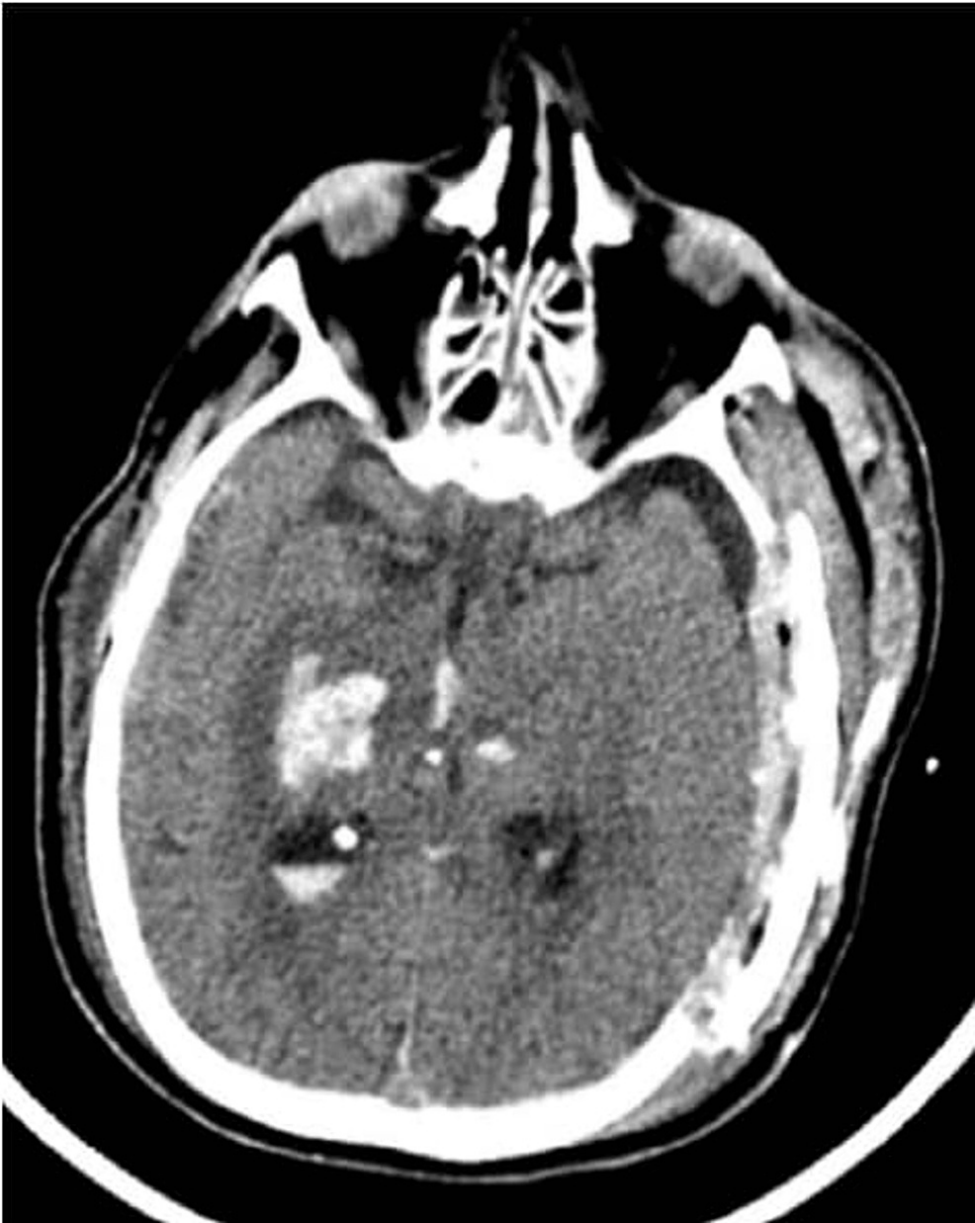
Axial NECT shows an intraparenchymal contusion in right capsulo-ganglionic region and intraventricular bleed noted with minimal mass effect compressing basal cisterns - Rotterdam score 3 NECT: non-contrast-enhanced computed tomography
